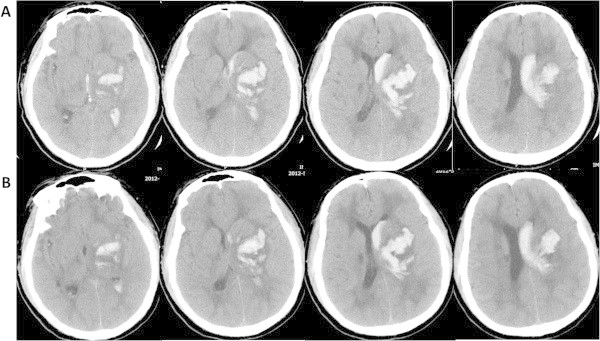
Head CT scan at the second admission. A. An acute irregular large hematoma presents near the left basal ganglia with a prominent mass effect, which extended into the ventricular system. B. CT scans three days later show no significant change of the size of hematoma.
